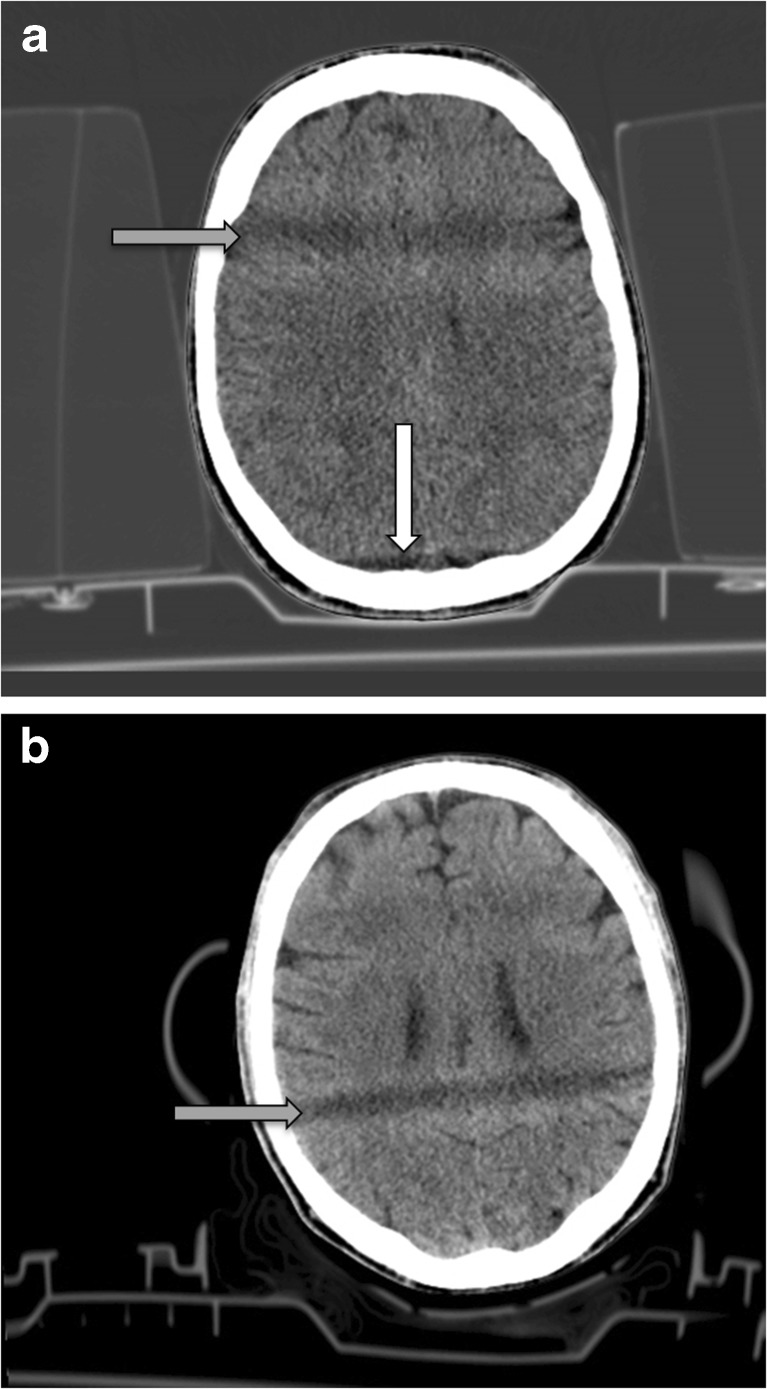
a Patient CT scan with artifact caused by headblocks. Set up indicates rigid spineboard plus soft foam headblocks. *Grey arrow* indicates hypodensity caused by material to air transition (earholes) in the headblocks. *White arrow* indicates line artifact cause by the base plate of the headblocks. b Patient CT scan with artifact caused by new design headblocks. Set-up indicates rigid spineboard plus new design headblocks. *Grey arrow* indicates shade caused by material to air transition in the headblocks
